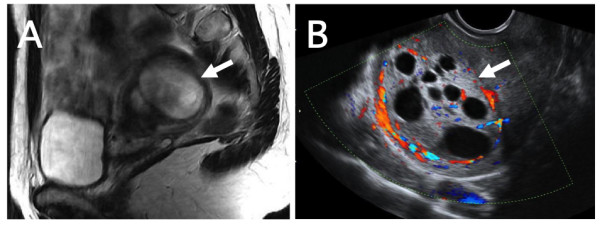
Diagnostic image findings of bizarre leiomyoma. (A) A sagittal T2-weighted magnetic resonance image of bizarre leiomyoma in the fundal portion of the uterine corpus. A heterogeneous ovoid tumor with an intensity signal that was mostly higher than that of the surrounding myometrium deformed the uterine cavity. (B) A transvaginal ultrasonography image of the uterine tumor with intratumoral multiple low-echoic cystic structures. Abundant blood flow around the tumor was noted.
